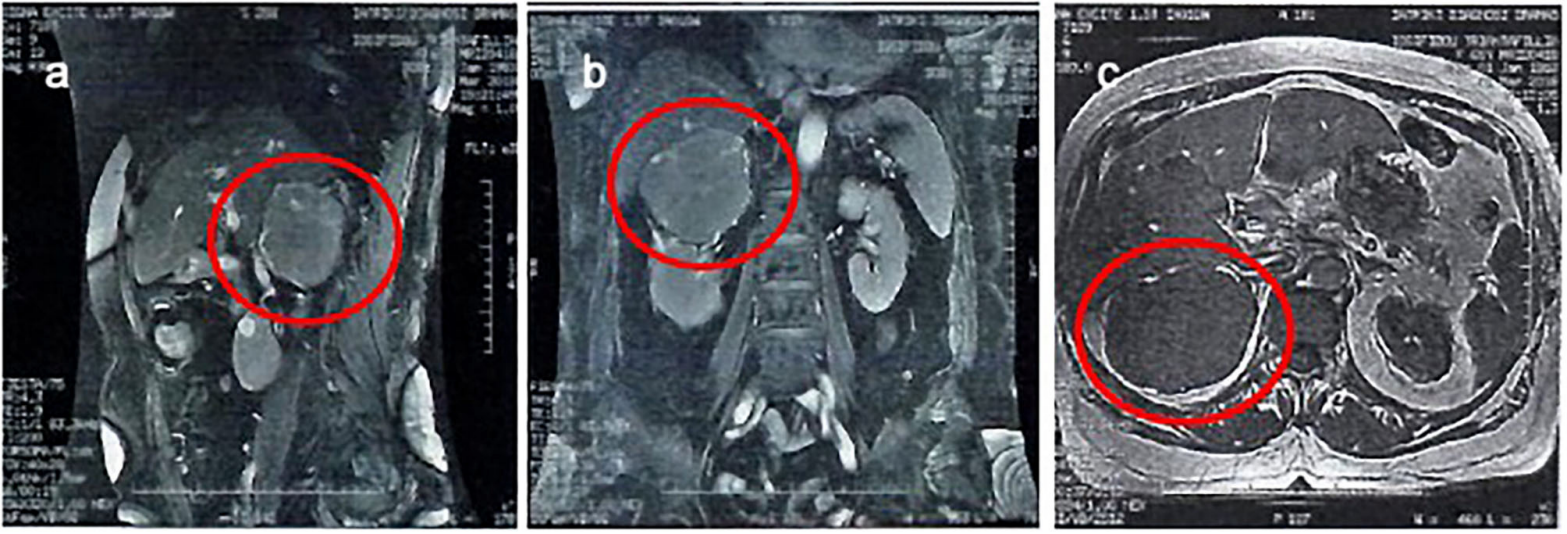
An abdominal MRI scan was performed, and it showed the right adrenal gland lesion. It also showed a clear distinction between the lesion and adjacent structures, without any infiltration to the inferior vena cava, or presence of pathological lymph nodes, nor were there any pathological lesions or enhancement in the liver. A sagittal T2 view (a), a coronal T2 view (b), and a transverse T1 view (c).
